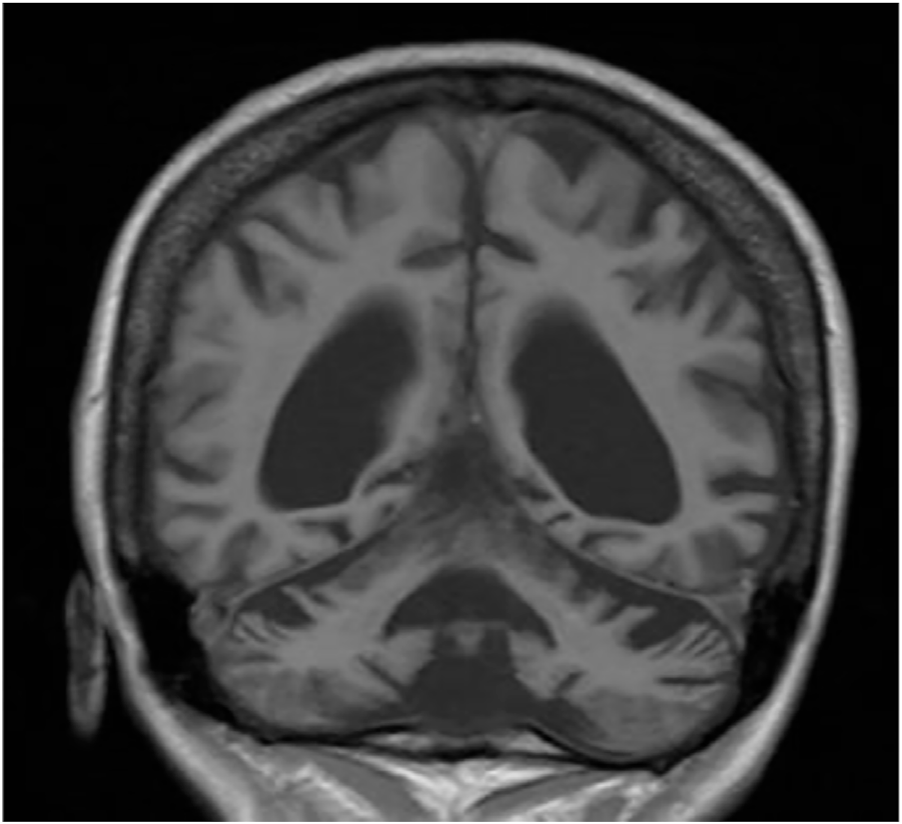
MRI—massive cortical and subcortical atrophy frontal section.
